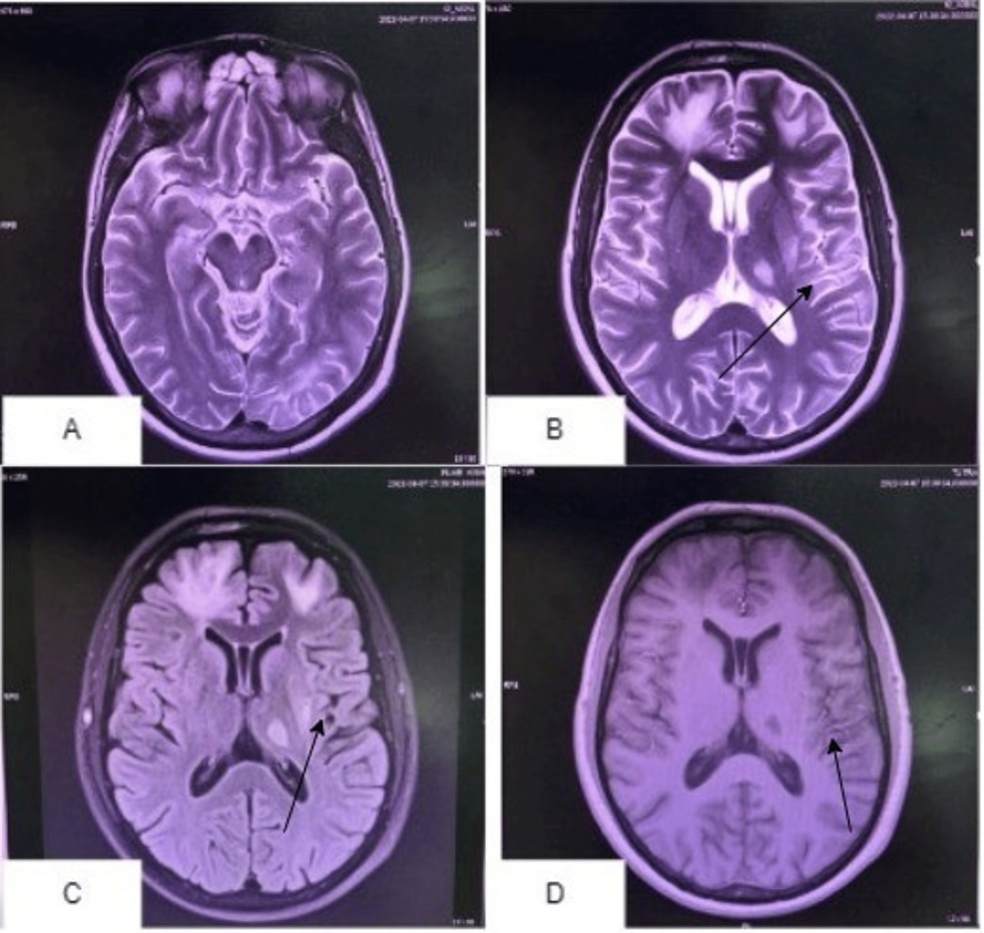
Brain multi-sequential MRI Multiplanar, multi-sequential MRI of the brain with and without contrast in images A, B, C, and D showing interval development of small T2 and FLAIR hyperintense signals noted in the left peri-insular region (C and D) and in the left medial temporal lobe (B).
